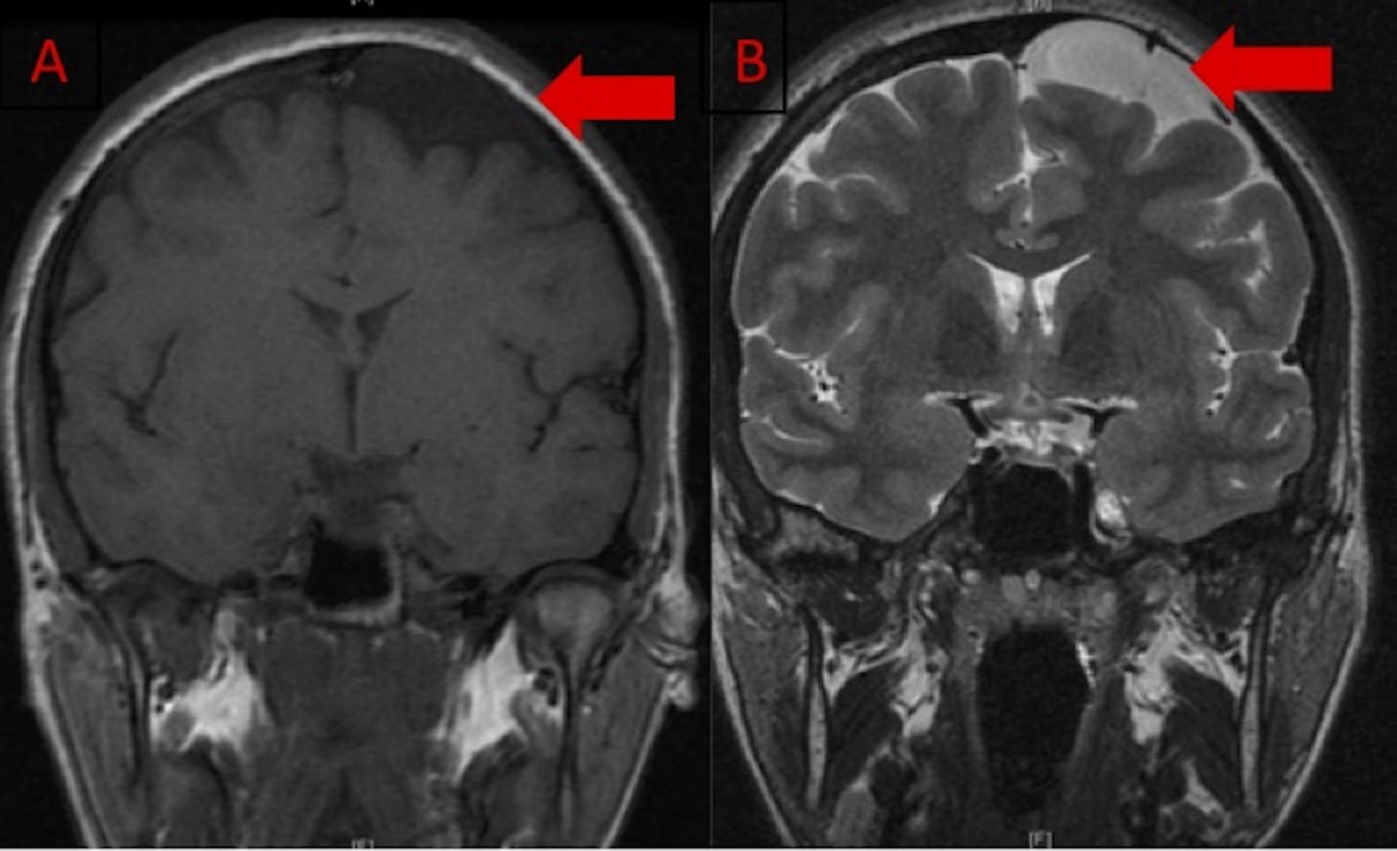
Magnetic resonance imaging of the brain without contrast MRI obtained two years prior to admission. A: Coronal T1-weighted image demonstrating a left frontal hypointense extra-axial lesion. B: Coronal T2-weighted image demonstrating a left frontal hyperintense extra-axial lesion. Both images are suggestive of an arachnoid cyst. MRI: Magnetic resonance imaging
